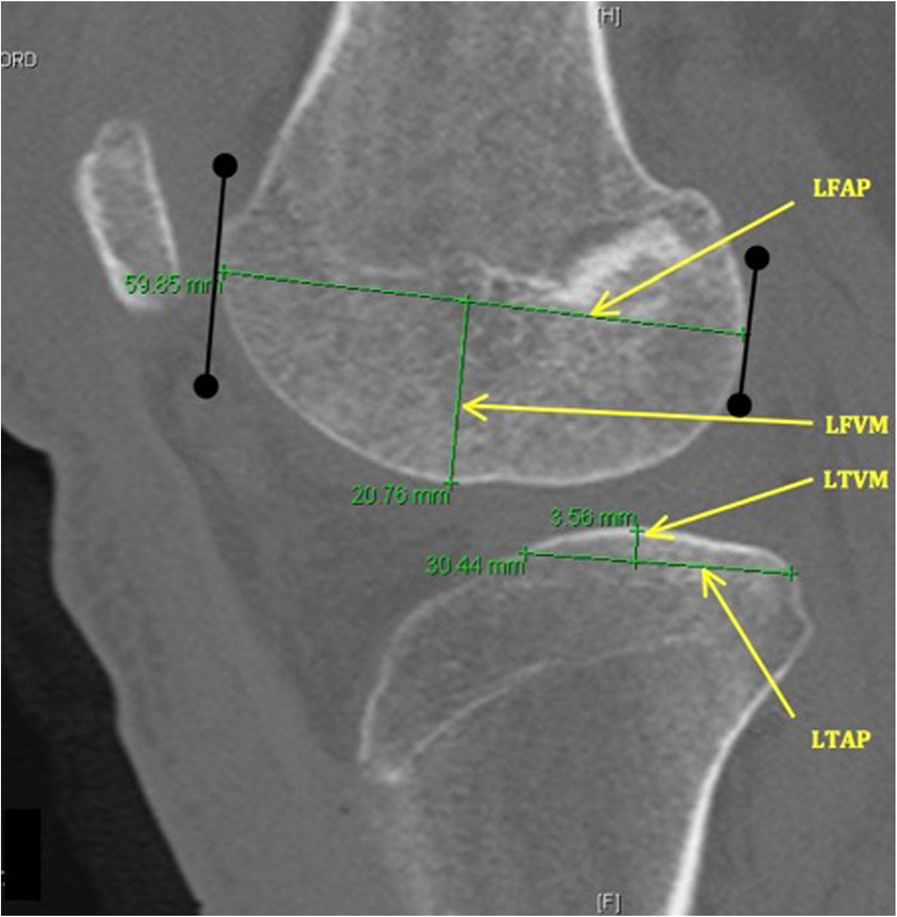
Measurements on a sagittal CT reconstruction of the lateral compartment. LFAP: lateral femoral condyle antero-posterior length. LFVM: lateral femoral vertical maximum of the curvature. LTAP: lateral tibial condyle antero-posterior length. LTVM: lateral tibial plateau vertical maximum of the curvature
